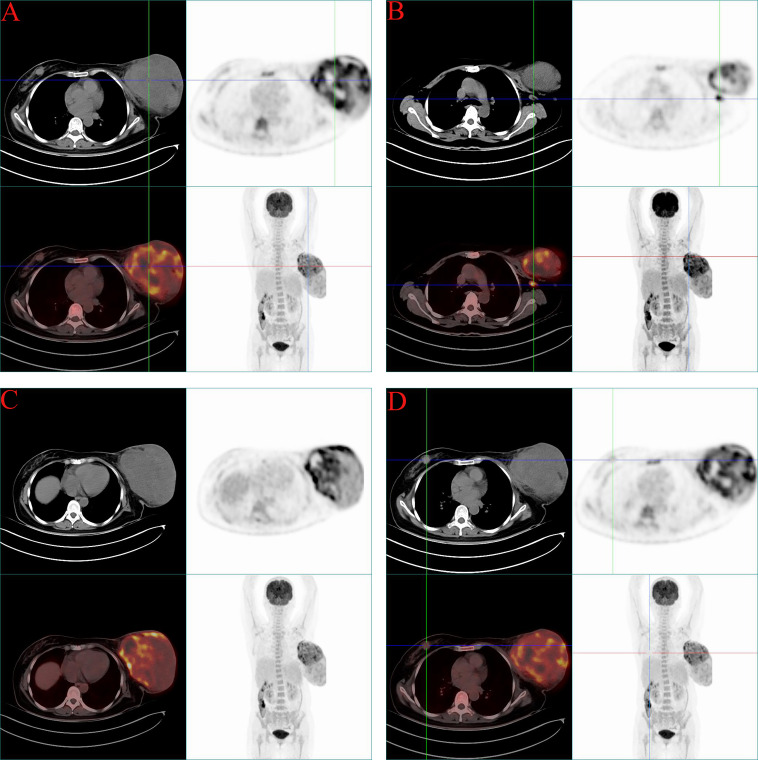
Representative ^18^F-FDG PET-CT images (A–D). Axial CT, PET, and fused PET/CT images show heterogeneous intense FDG uptake within the left breast mass and markedly FDG-avid ipsilateral axillary lymph nodes. Whole-body maximum-intensity-projection images show no FDG-avid distant metastases.
